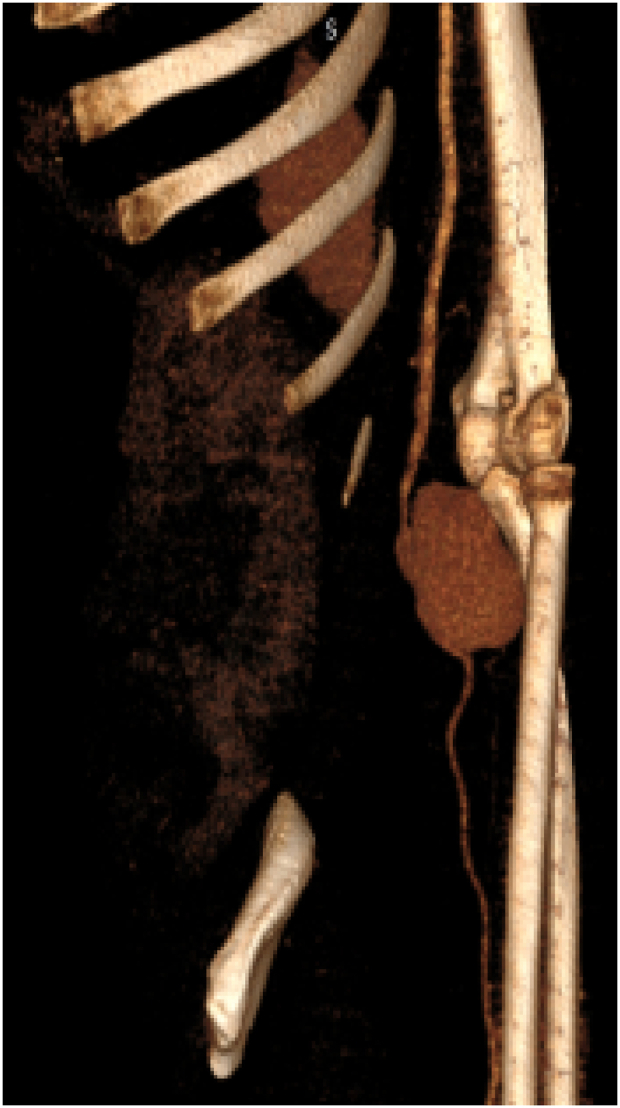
CT-Angiography with 3D-reconstruction image demonstrating Radial artery pseudoaneurysm.
